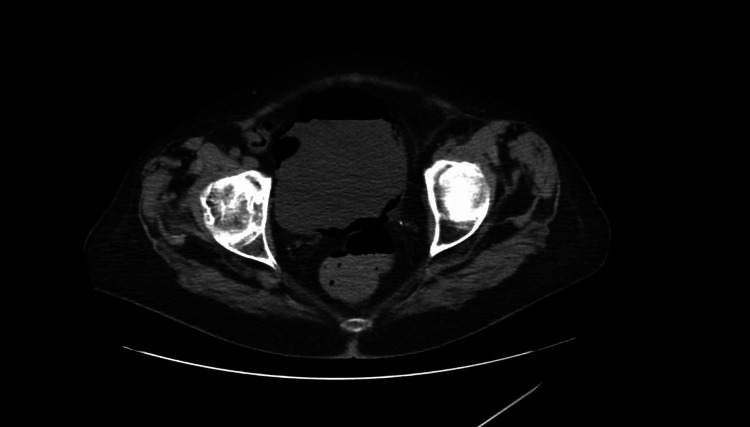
CT scan of the pelvis done after eight days of antibiotics showing improvement in the air within the bladder wall
